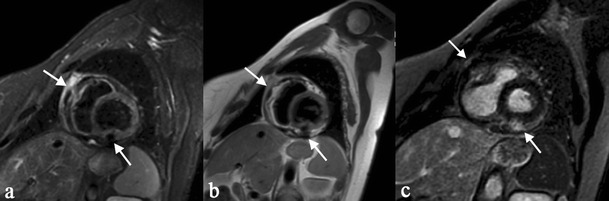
Cardiac MRI involvement in a patient with systemic sarcoidosis. Triple inversion recovery (Fig. 22a) and double-inversion recovery sequences (Fig. 22b), both obtained on short axis views, clearly show hypointense calcified myocardial and pericardial nodules (*white arrows*). After gadolinium administration, foci of delayed enhancement (*white arrows*), involving also the pericardium, are recognizable (Fig. 22c)
